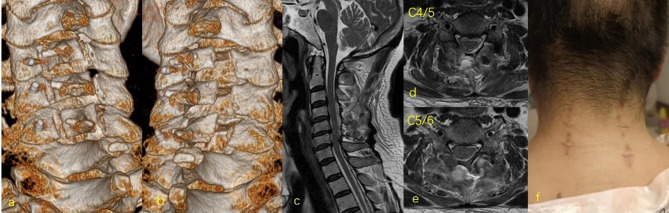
Postoperative 3D CT of C4-C6 showed widening of the spinal canal after BBE laminoplasty using suture anchor fixation (A,B) Postoperative MRI showed full decompression of spinal cord with an adequate subarachnoid space (C–E). Six wounds were created for the cervical laminoplasty at three levels (F).
